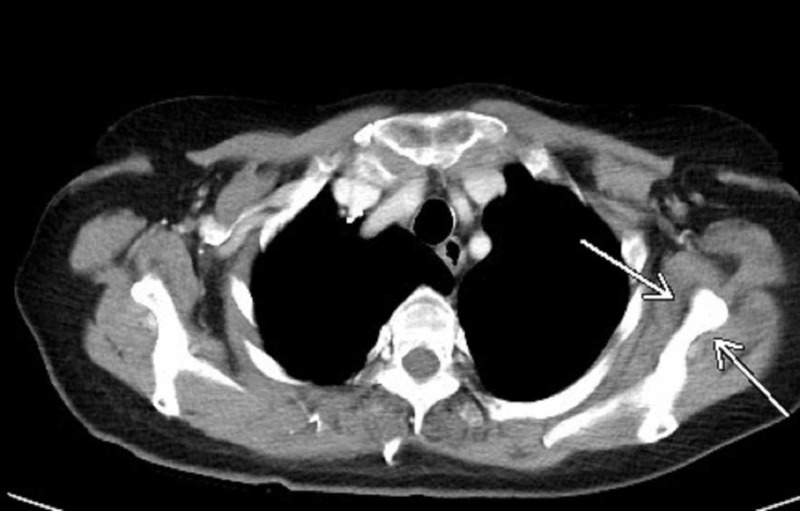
CT chest showing resolution of the lytic expansile scapular lesion, after three years of treatment with nivolumab.
